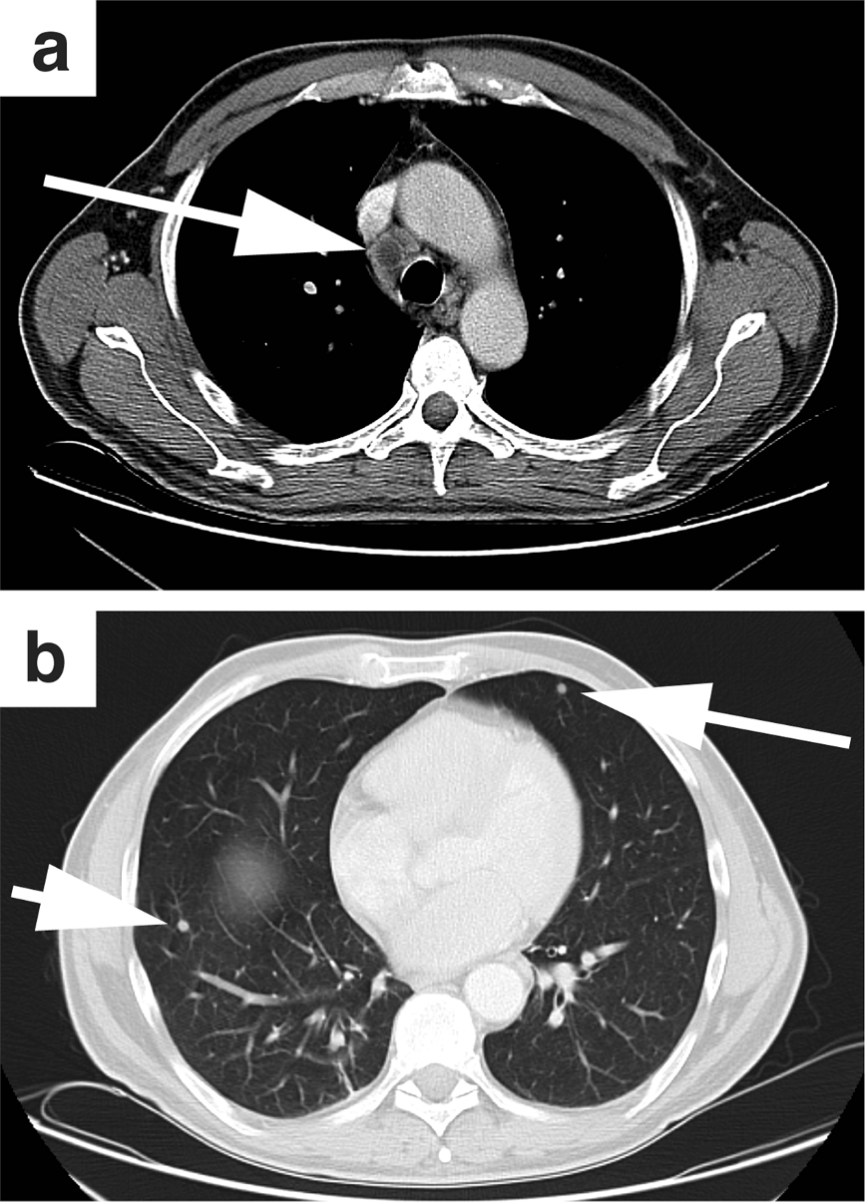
Radiological diagnosis of disseminated tuberculosis. CT scan of the thorax on day +43 after allogeneic stem cell transplantation revealed a borderline sized mediastinal lymph node with central necrosis (a) as well as multiple pulmonary nodules (b).
